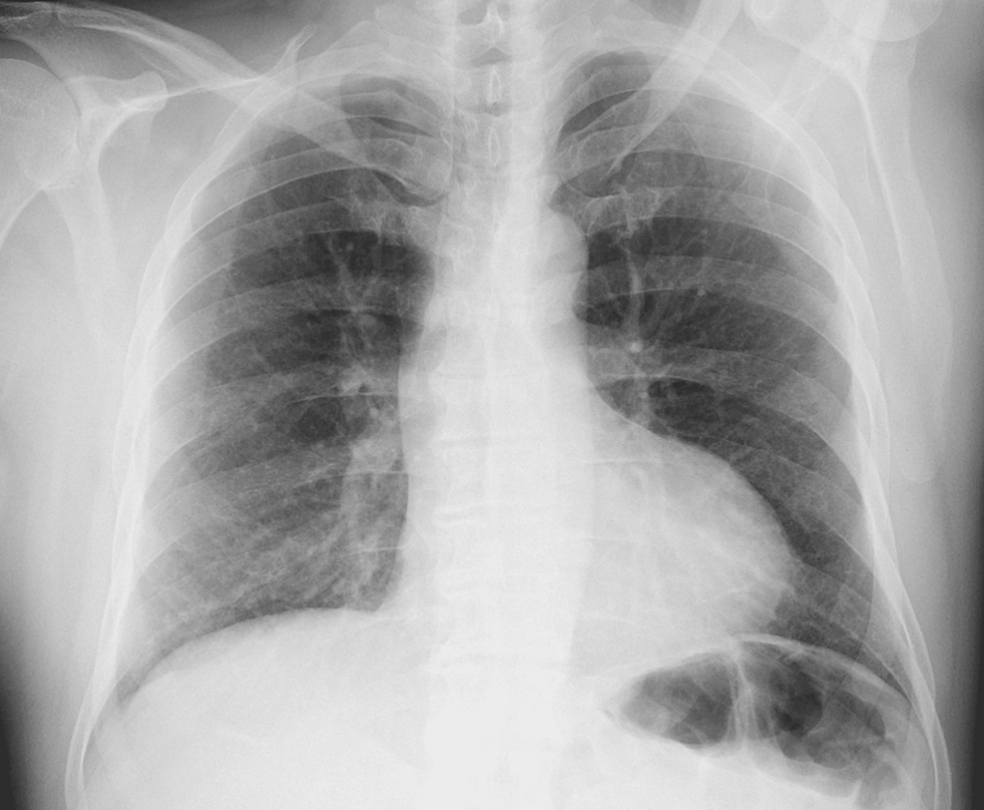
PA X-ray of the chest showed no consolidations and no infiltrations; a cardiac silhouette is observed with a cardiothoracic index of 0.49, and costophrenic and cardiophrenic angles visible. PA: posteroanterior
